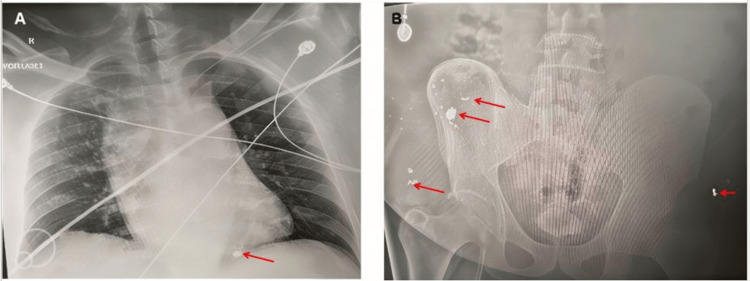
Radiographic imaging taken at trauma bay (A) Portable chest X-ray showing wide mediastinum and projectile lodged in heart, marked by a red arrow. (B) Pelvic X-ray showing the majority of bullet fragments projecting along the right wing of the ilium, labeled by red arrows. Red arrows on A and B pointing towards bullet fragments in chest X-ray and pelvic X-ray.
